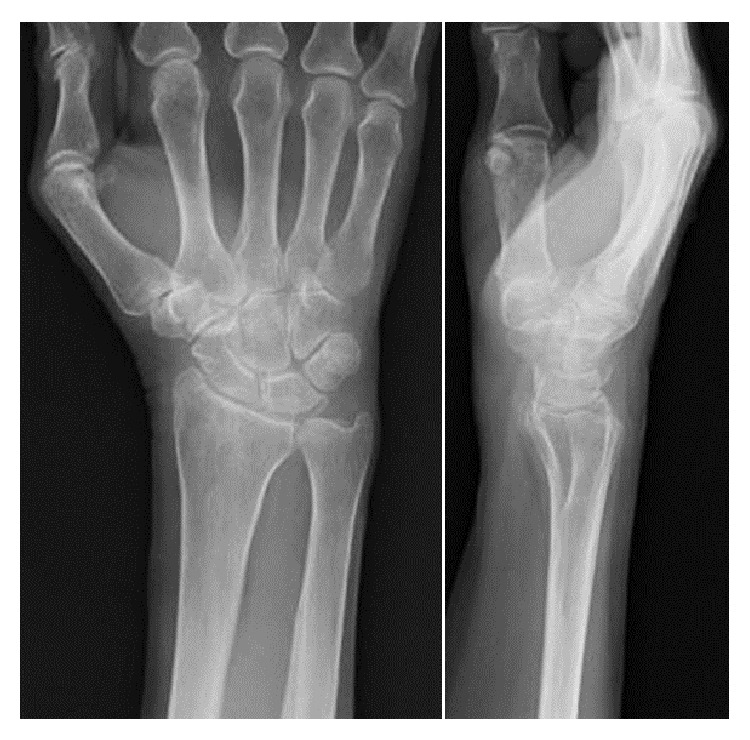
X-ray findings at last follow-up, 6 months after surgery.
